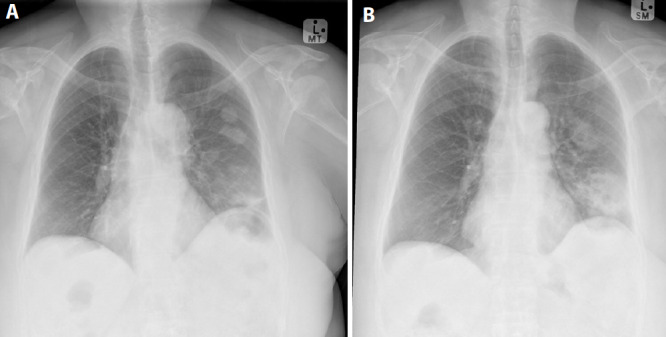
Initial (A) and repeat (B) chest radiograph.
